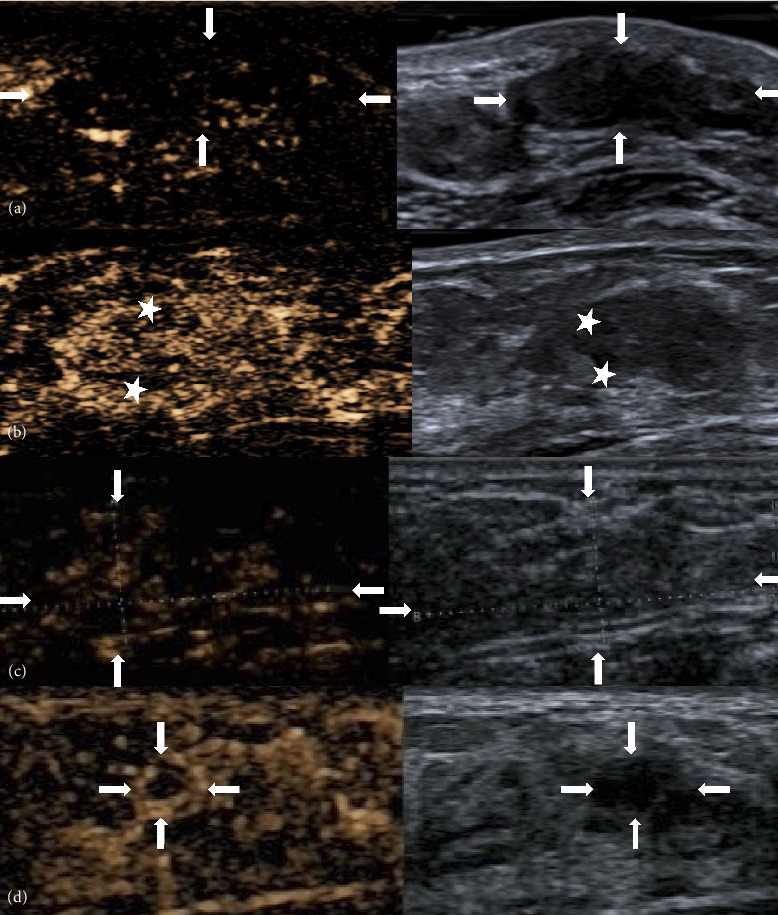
(a) CEUS demonstrates the lesion as having low enhancement, with uneven perfusion showing a low perfusion pattern. After enhancement, the lesion exhibits clear boundaries and regular morphology (as indicated by “↑”); (b) CEUS reveals uneven enhancement with perfusion defects, presenting as patchy perfusion defects (depicted by “★”), the defective perfusion areas correspond to the cystic hypoechoic regions (indicated by “★”), and after enhancement, the lesion demonstrates clear boundaries and regular morphology; and (c) conventional ultrasound indicates BI-RADS 4b, while CEUS reveals low enhancement with internal perfusion defects. CEUS findings suggest BI-RADS 4a (as indicated by “↑”). (d) Conventional ultrasound suggests BI-RADS 4c, while CEUS reveals peripheral high enhancement, central low enhancement, uneven contrast agent perfusion, clear boundaries, and regular morphology. CEUS findings suggest BI-RADS 3 (as indicated by “↑”).
